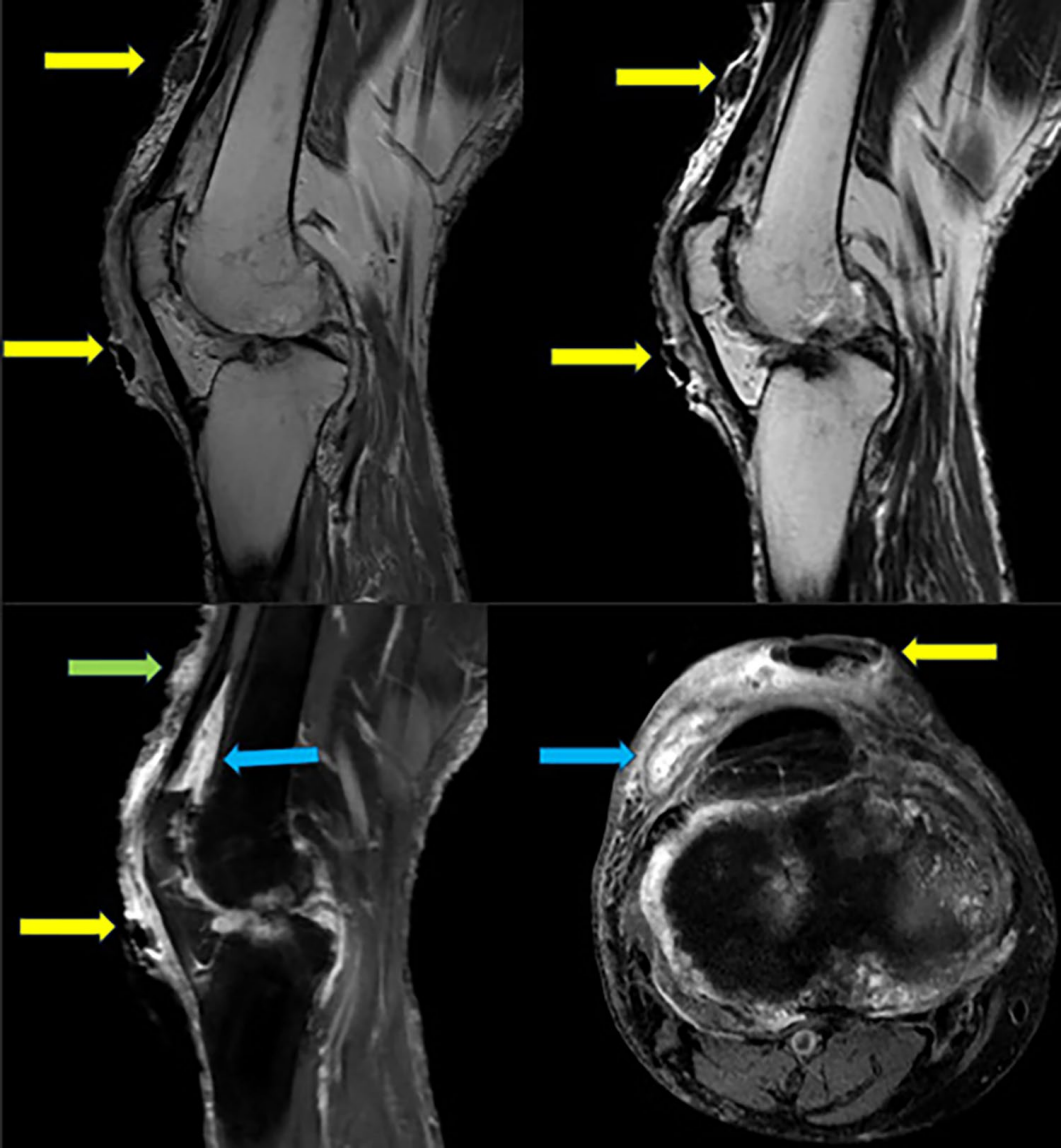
Magnetic resonance images of the right knee, sagittal proton density, T2, T1 with contrast and SPAIR axial slices, of the patient described in Figure 1, where the long yellow arrows show low signal nodules without contrast enhancement located in the prepatellar soft tissues, and in particular one with contrast enhancement in the prefemoral region (long green arrow). The blue arrows show thickening and enhancement of the synovial membrane of the suprapatellar bursa with internal particulate effusion. Findings compatible with subcutaneous gouty tophi and findings due to acute synovitis
